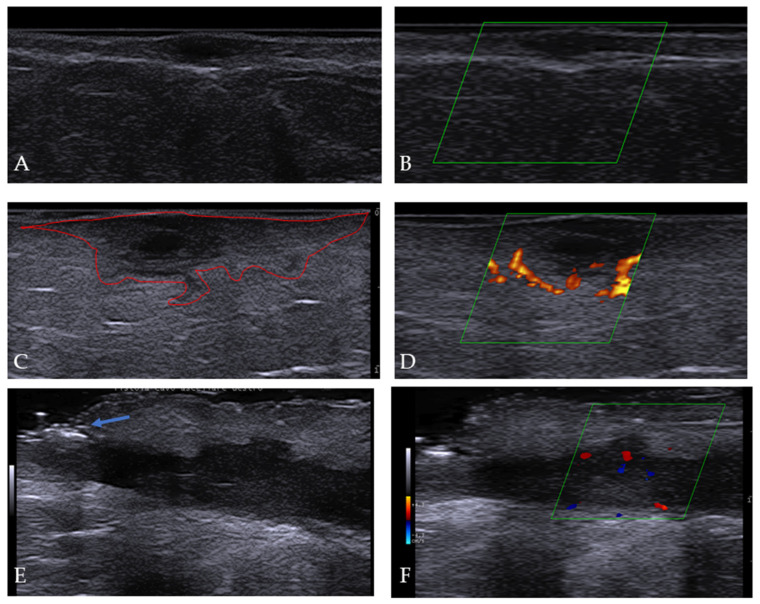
Cutaneous scans of areas affected by HS with 18 mHz probe revealed the presence of: (A) A nodule that presented as a round anechoic nodular dermal lesion. (B) Absence of power doppler signal as in a non-inflammatory nodule. The lesion was the result of a treated abscess with topical clindamycin and it was stable through 12 weeks of follow-up with twice-weekly topical clindamycin application. Lesion was surgically excised thereafter. (C) Abscess displaying as a hypoechoic/anechoic fluid deposit with a polypoid aspect and connection to the base of a widened hair follicle. (D) Power doppler study revealed a peripheral signal corresponding to the polypoid extension of the periphery of the lesion. (E) A tunnel characterized by an anechoic/ipoechoic band-like structure in the dermis/hypodermis and connected to the base of a widened hair follicle (blue arrow). (F) Peri-intra power doppler signal of the tunnel.
